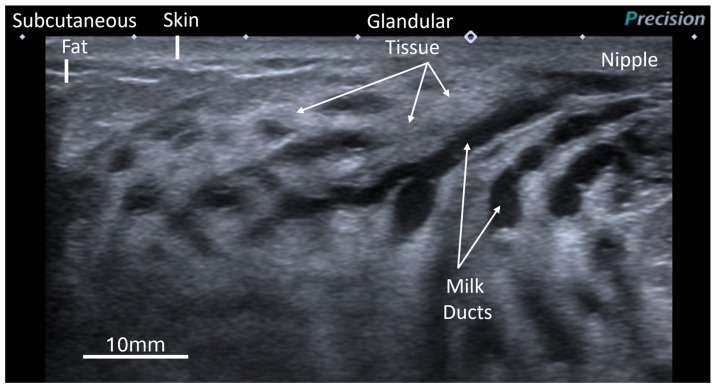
Ultrasound image of the lactating breast showing main milk ducts (dark, hypoechoic) within the breast, under the areola. Glandular tissue is hyperechoic and the fat more hypoechoic compared to the glandular tissue.
